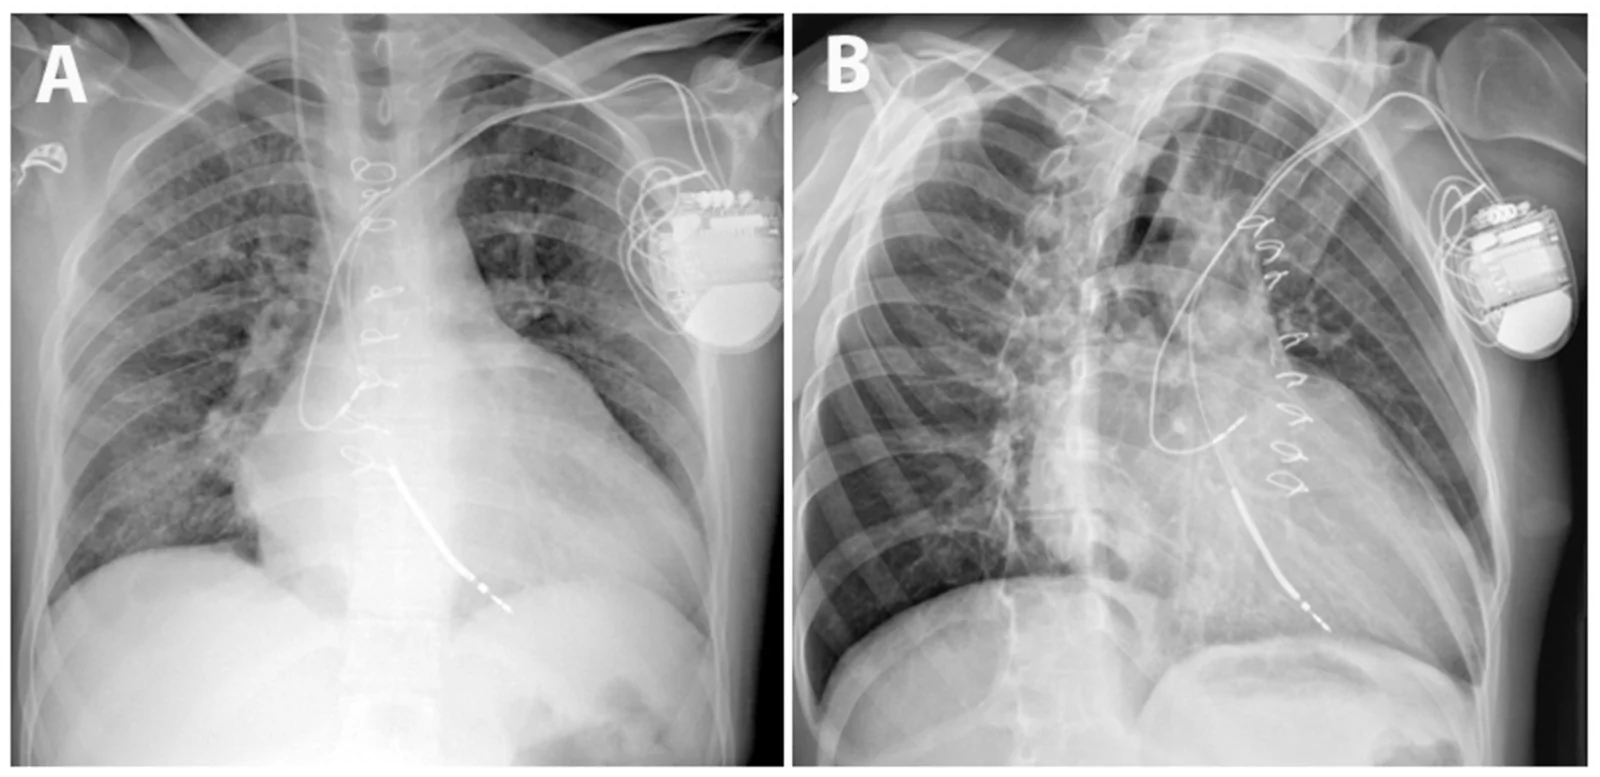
Preprocedural chest radiographs. (A) Frontal chest radiograph demonstrating cardiomegaly with pulmonary vascular congestion in the setting of acute decompensated heart failure. (B) Right anterior oblique (RAO) 60° projection obtained showing the left-sided dual-chamber implantable cardioverter-defibrillator (ICD) and median sternotomy wires from previous cardiac (septal myectomy).
